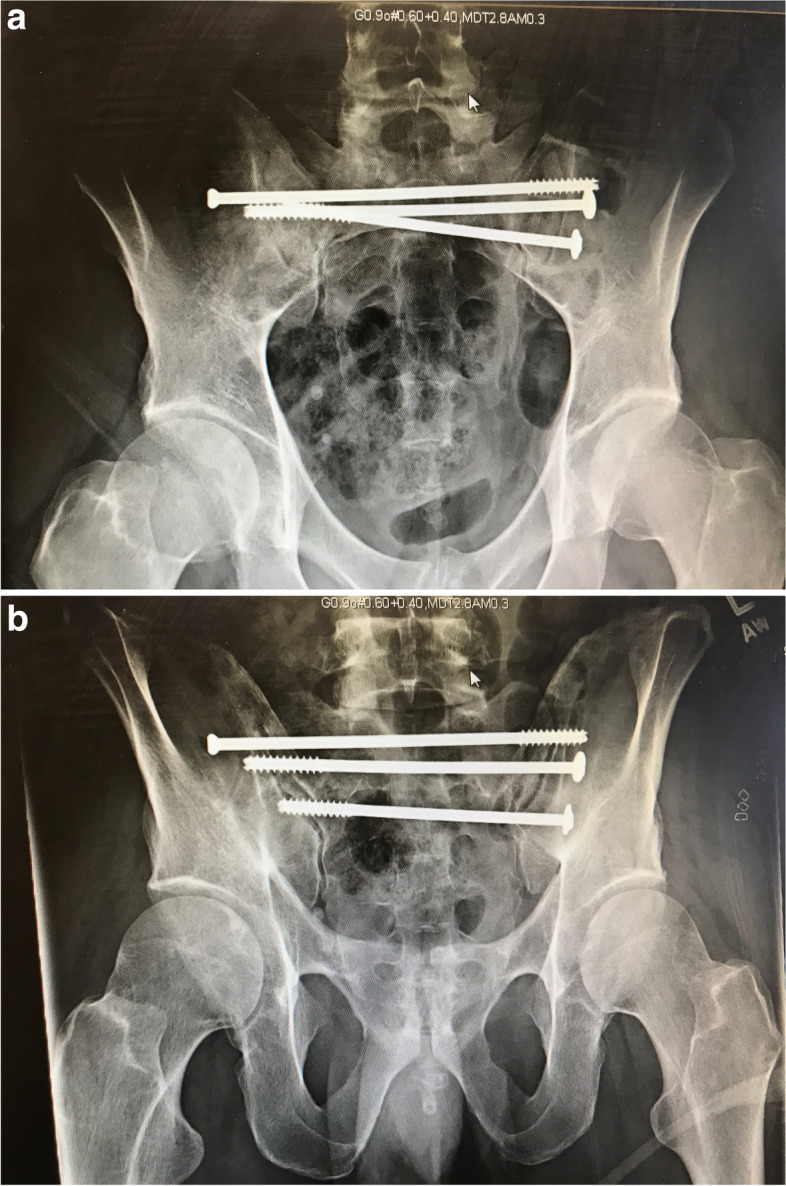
Post-operative pelvic radiographs (A-B) of a posterior pelvic ring injury fixed with three trans-sacral screws in both S1 and S2
